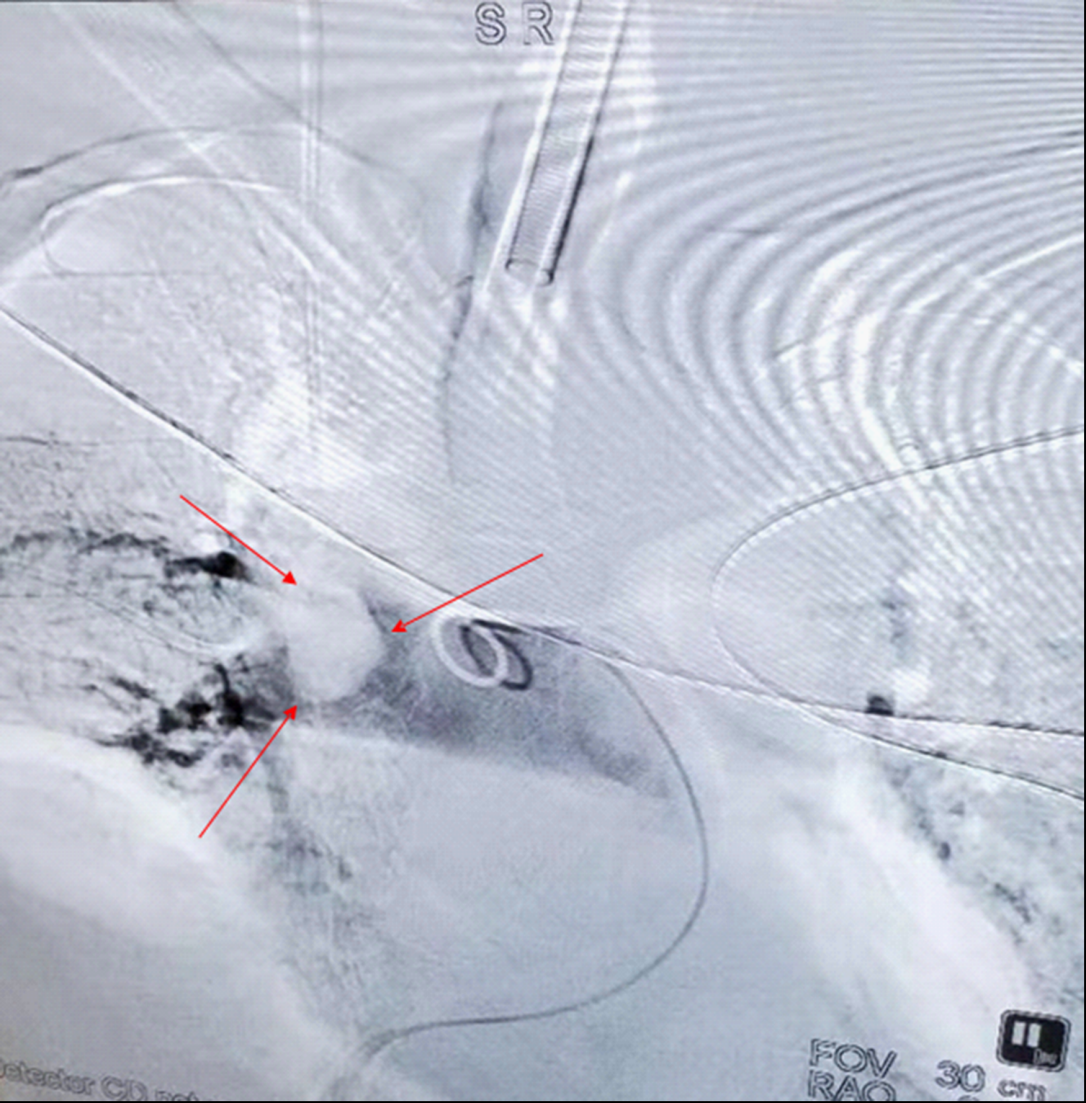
Computed tomography angiography of the lung approximately 3 h after hemodynamic fluctuations occurred: multiple embolisms in the two main pulmonary arteries and bilateral pulmonary artery branches. The arrows denote a very large embolus.
